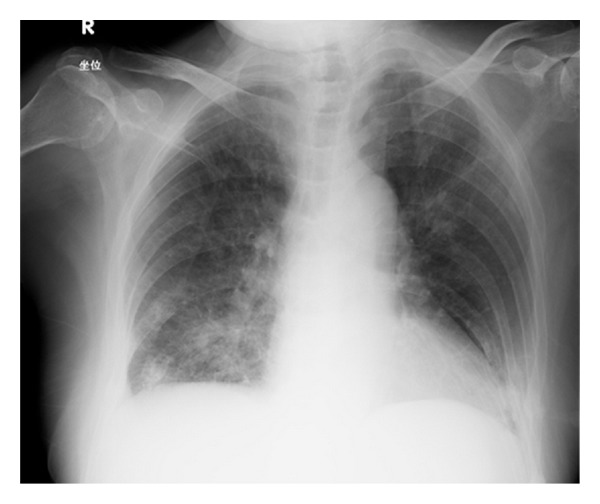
Chest radiograph revealed bilateral ground-glass infiltrates and reticular shadows.
